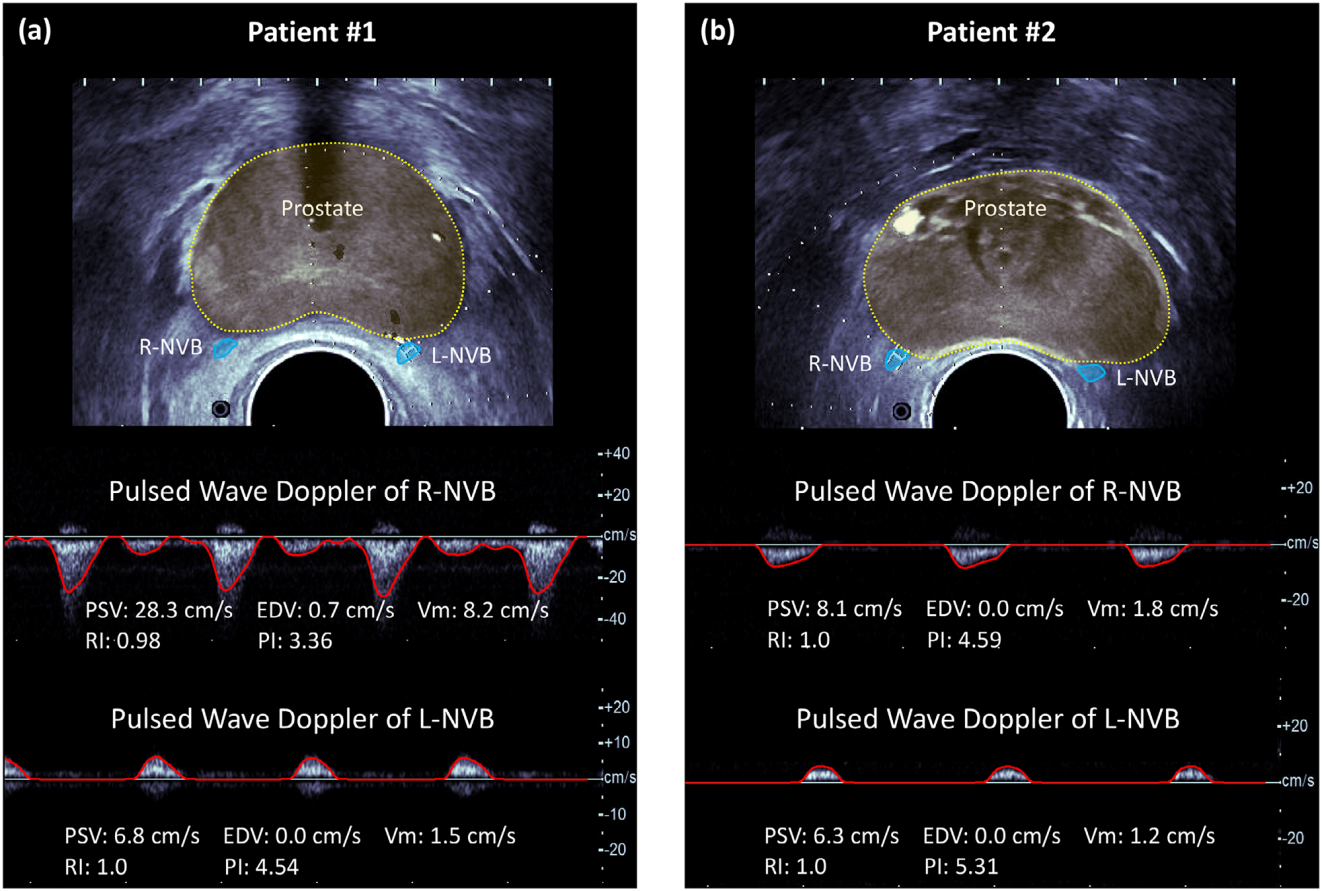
Ultrasound measurements for two patients: (a) patient #1 with normal erectile function (EPIC‐CP score = 0) and (b) patient #2 with moderate ED (EPIC‐CP score = 6). The upper panels show B‐mode images of the prostate gland with NVBs highlighted on the right (R‐NVB).
